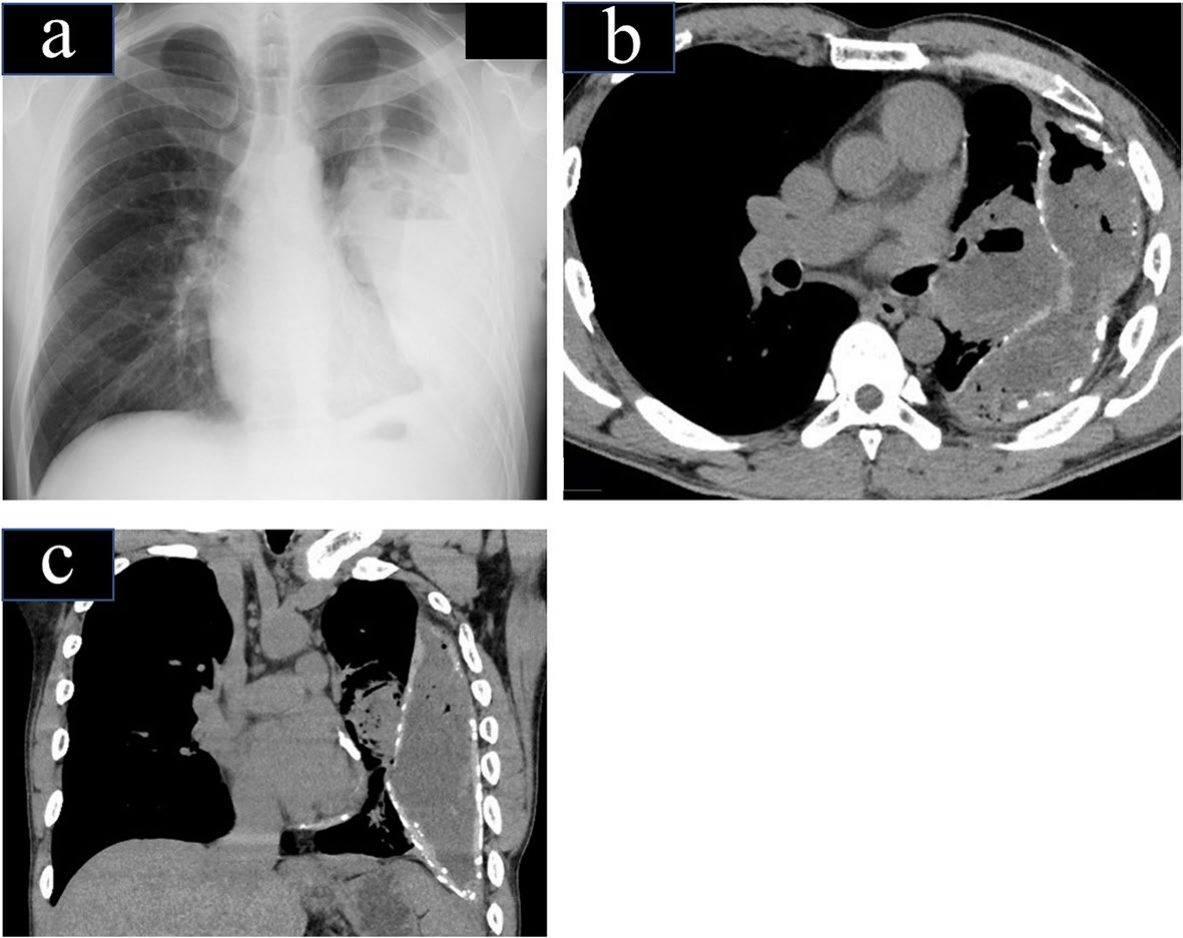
Imaging findings on admission. a Chest X-ray findings on admission showed pleural effusion from the left middle lung field to the lower lung field, including air–fluid levels. b Chest CT (horizontal section) revealed an extrapulmonary lesion with fluid and gas inside, accompanied by calcification in the surrounding area. c In a coronal section, extrapulmonary lesions were observed from the second rib to the diaphragm
